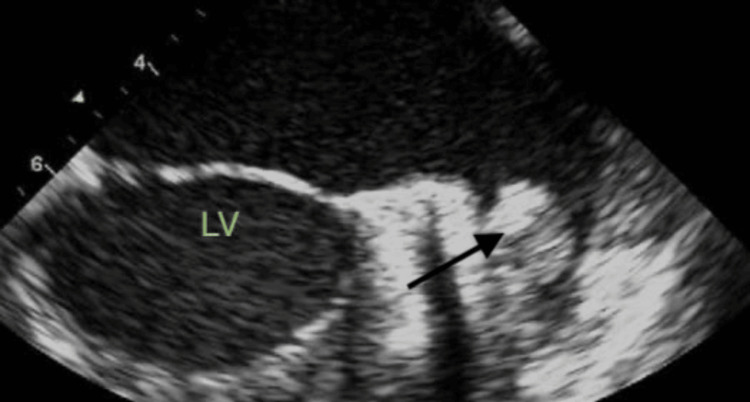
Transesophageal echocardiogram Black arrow showing thrombus in the left atrial appendage. LV: left ventricle.
